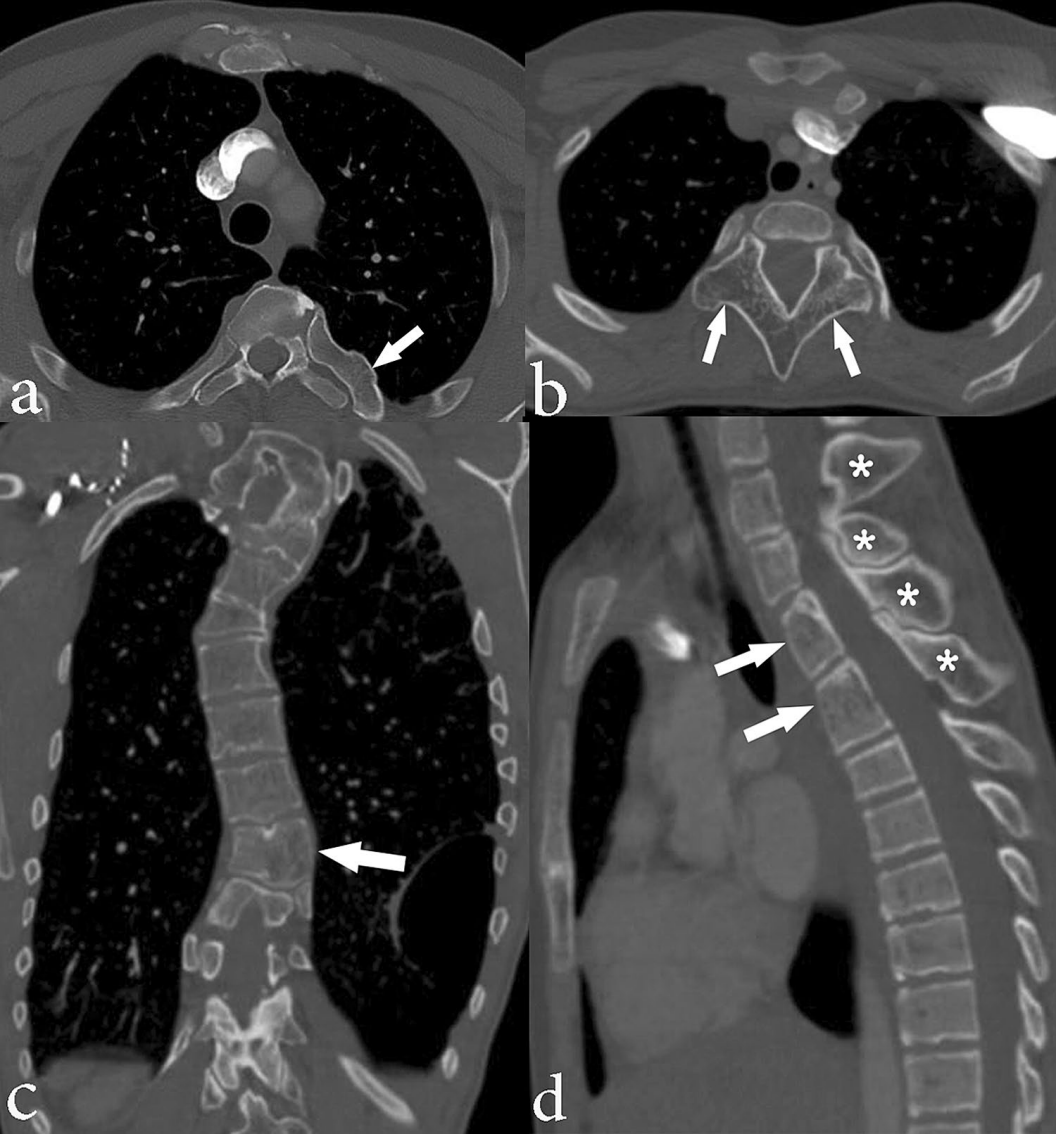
Chest CT images depicting skeletal abnormalities in individuals with Proteus syndrome. Axial CT image of the chest in bone windows shows (a) overgrowth of the left fifth posterior rib (arrow) in a 61-year-old male (note normal size of the contralateral right posterior fifth rib) and (b) overgrowth of the posterior elements of the spine (arrows) in a 22-year-old female. (c) Coronal CT image in bone windows in a 36-year-old male shows scoliosis of the thoracic spine. There is asymmetric overgrowth of multiple vertebral bodies resulting in asymmetric vertebral body heights (example depicted by the arrow). (d) Sagittal CT image of the spine in bone windows in a 22-year-old female shows overgrowth of several spinous processes (asterisks) and several upper vertebral bodies (arrows).
